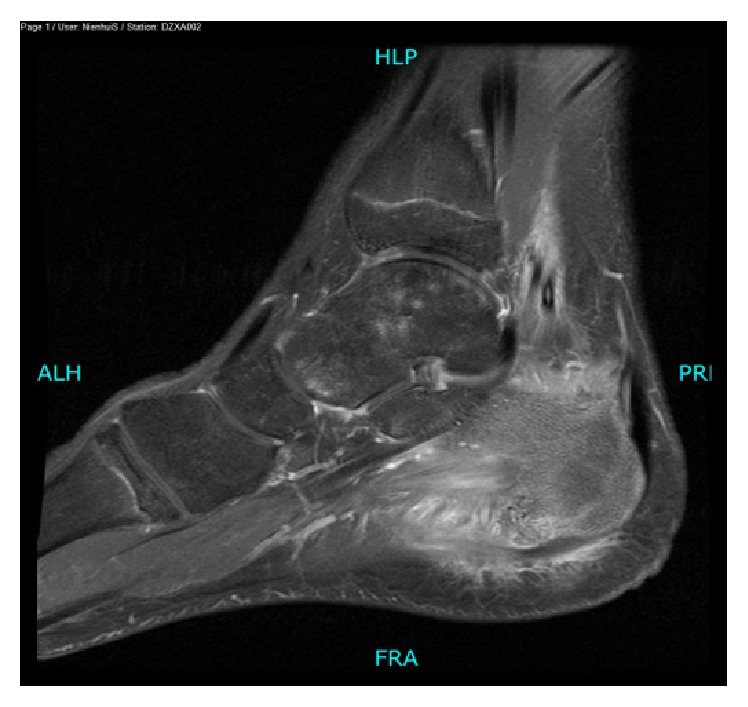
MRI T1 fat suppression after gadolinium: sagittal view of the left foot: attenuation of soft tissue near the enthesophyte.
